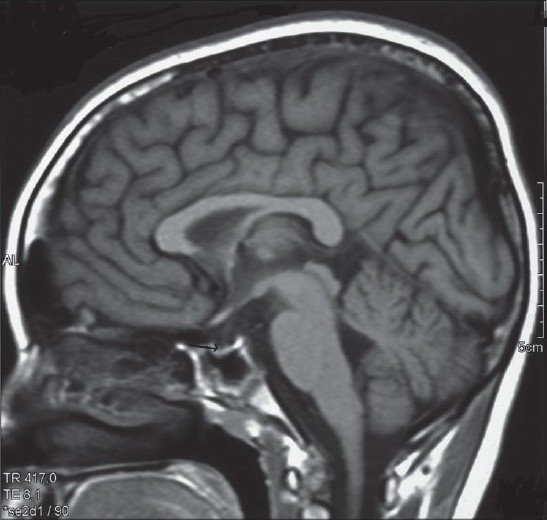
T1 sagittal magnetic resonance imaging showing reduced size of anterior pituitary gland (arrow)
